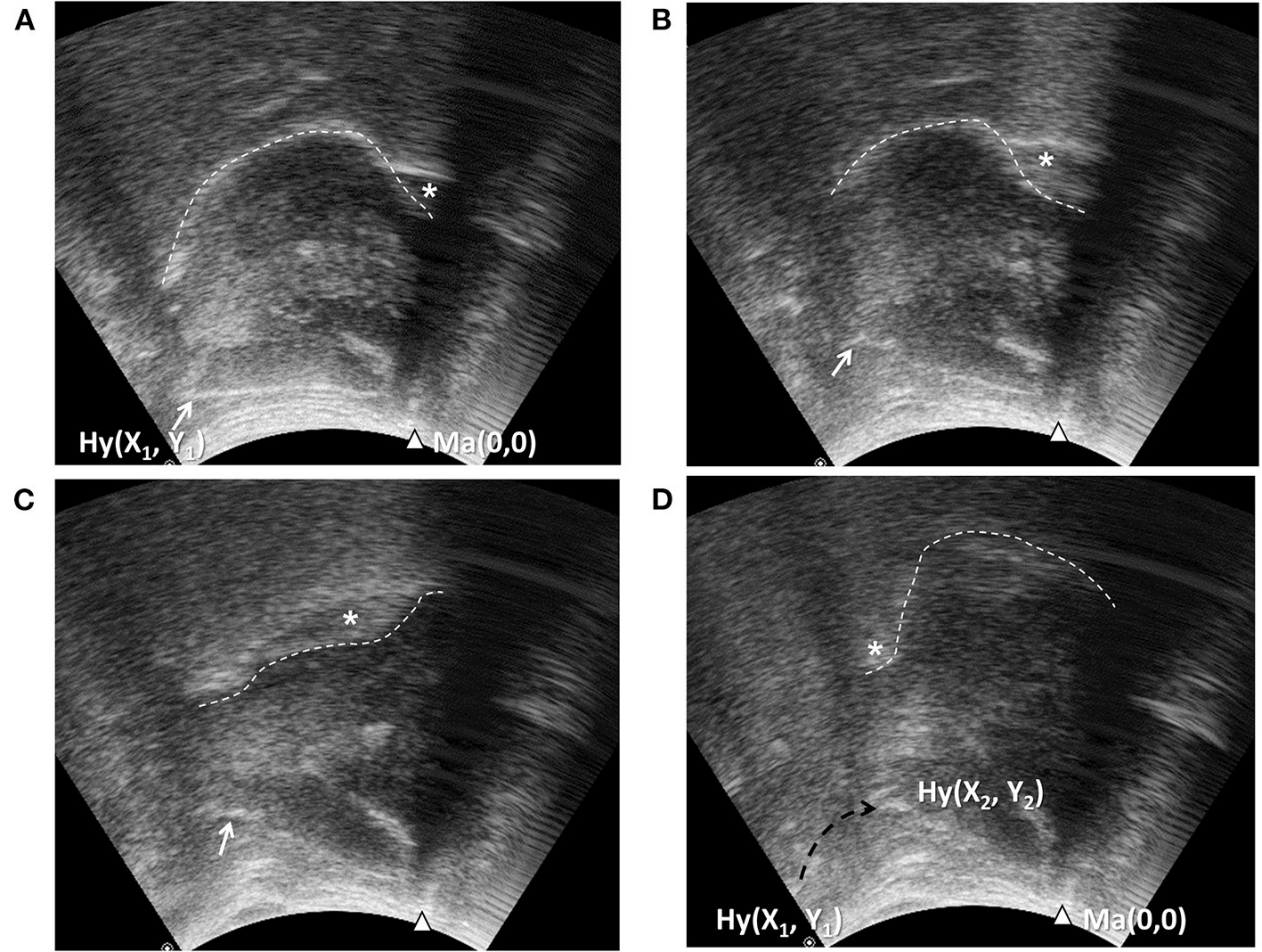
Ultrasonography assessment of tongue movement and hyoid bone displacement. Submental midsagittal ultrasonography (Figure 1A) showing the hyoid bone (arrow, Hy) at resting position (A), during excursion (B,C), and at maximal displacement (D). The position of the mandible (arrow head, Ma) in each frame was used as the reference point to calculate the position coordinates of the hyoid bone. X_1_, Y_1_, position of the hyoid bone at resting position; X_2_, Y_2_, position of the hyoid bone at maximal displacement; dashed arrow: trajectory of the hyoid bone. Wave-like movement of tongue (dashed line) propagating the bolus backward could be observed simultaneously.
